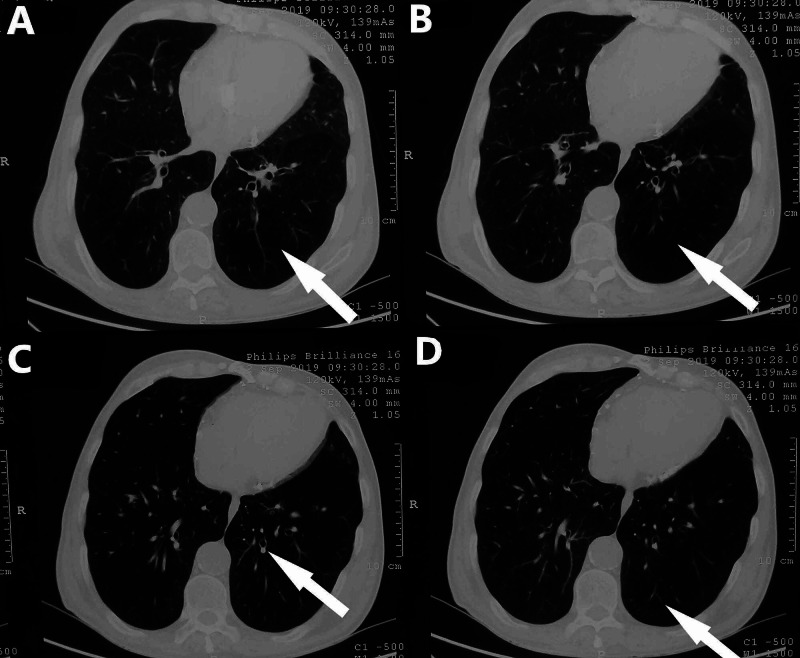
Computed tomography of the chest A, B: Arrows show reduced density in the left lower lobe. C, D: Arrows show bronchiectasis in the left lower lobe.
